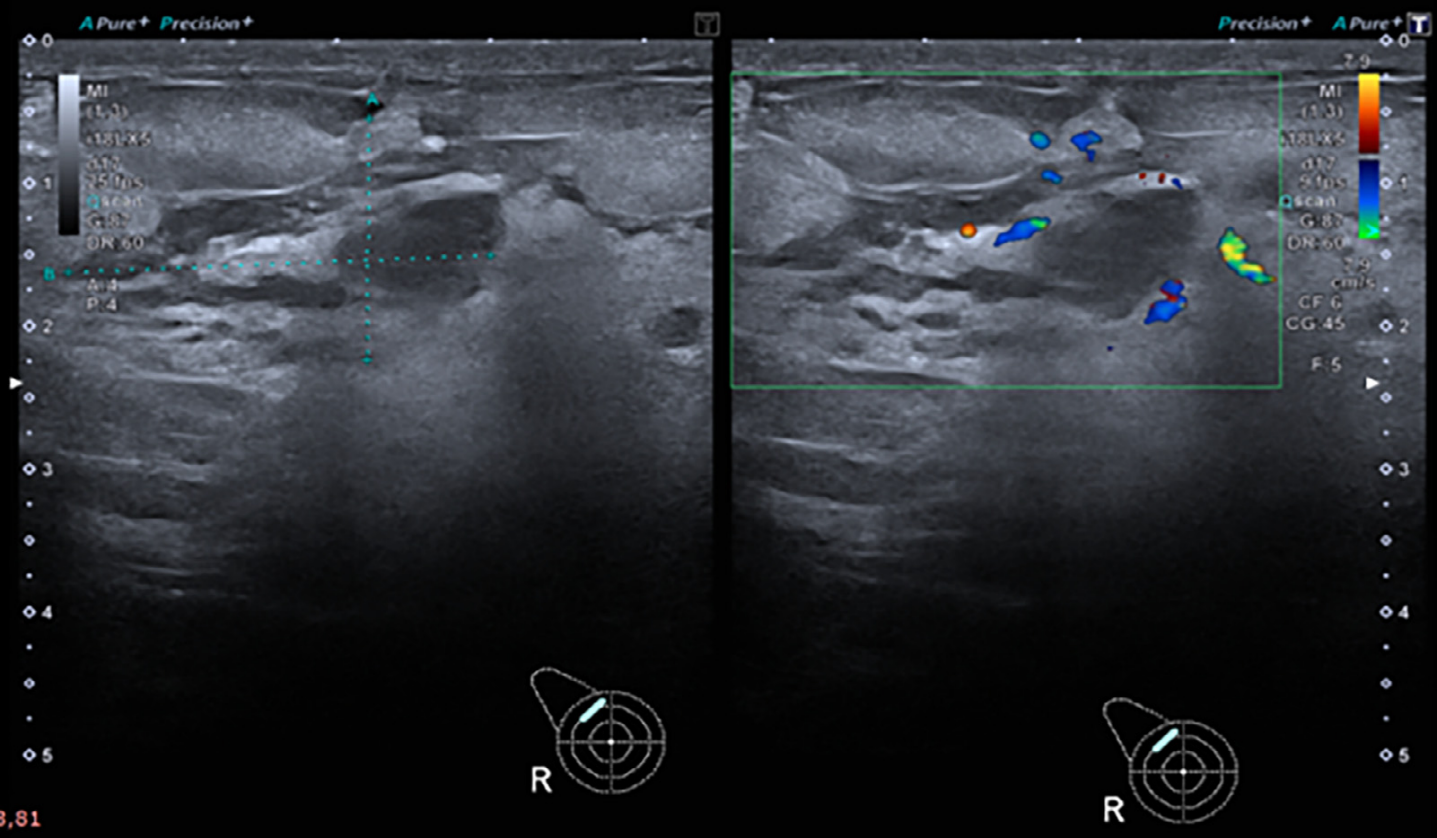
Ultrasound images showing ill-defined areas of abnormal, mixed hyperechogenic and hypoechogenic regions without a discrete mass in upper outer quadrant. Color Doppler shows hypervascularity.
